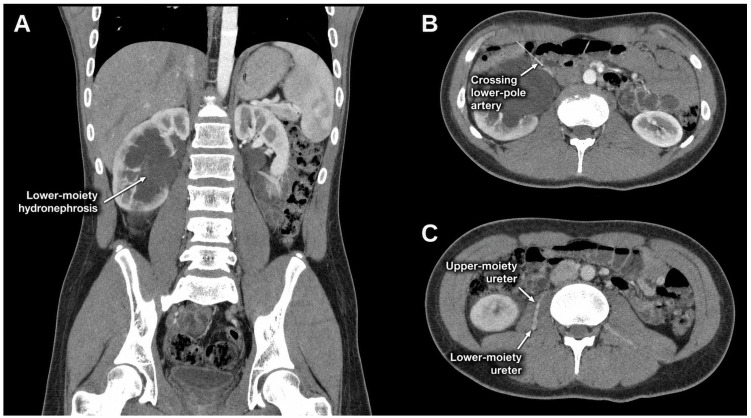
Preoperative CT urography of the right urinary tract. Representative CTU images demonstrated: (A) coronal delayed urographic-phase image showing hydronephrosis confined to the lower moiety with delayed excretion; (B) axial nephrographic-phase image showing a crossing lower-pole renal artery at the level of the lower-moiety ureteropelvic junction; the accompanying lower-pole vein was identified on the CTU series and confirmed intraoperatively; and (C) axial image demonstrating incompletely duplicated/bifid ureters. CTU localized the obstruction to the lower-moiety UPJ and showed no urolithiasis.
